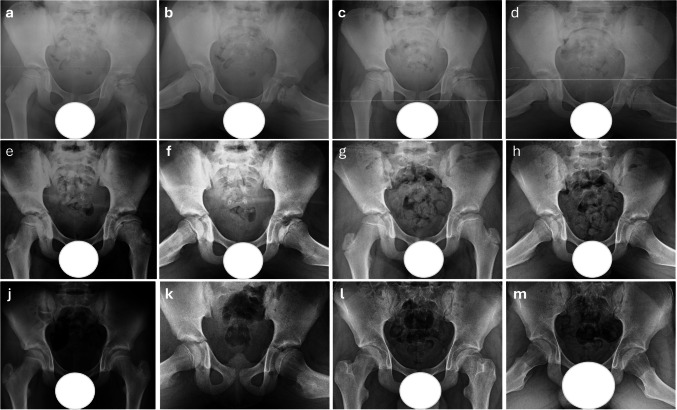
Serial radiographic follow-up of an 8-year-old male patient with Legg-Calvé-Perthes disease treated conservatively. Initial anteroposterior (AP) and frog-leg lateral hip radiographs at the time of diagnosis (8 years old), demonstrating Waldenström stage 3 (early reossification stage) with lateral subluxation and multiple"head at risk"signs, including metaphyseal cysts, Gage sign, and calcification in the lateral column (a, b). Radiographs at the 1-year follow-up, showing ongoing reossification with partial femoral head remodeling, though subluxation persists (c, d). Radiographs at the 3-year follow-up, indicating remodeling phase, with continued femoral head reshaping and improved acetabular containment. However, residual deformity remains evident (e, f). Radiographs at the 5-year follow-up, demonstrating remodeling phase with further improvement in femoral head sphericity and acetabular congruence (g, h). Radiographs at the 6-year follow-up, showing excellent femoral head remodeling, with near-complete restoration of sphericity and improved hip joint congruency (j, k). Radiographs at the 8-year follow-up, demonstrating complete remodeling, with the resolution of lateral subluxation. The femoral head has achieved *Stulberg Class I* morphology, with a subluxation ratio of 1, indicating full containment and joint congruency (l, m)
